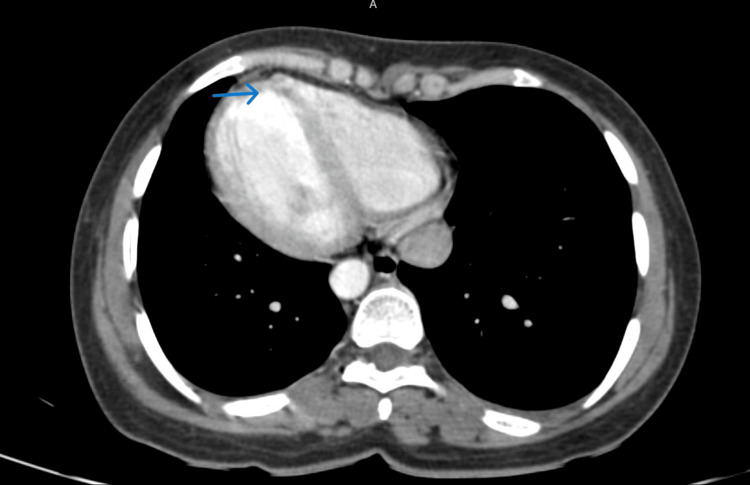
Axial late arterial phase contrast CT scan of the abdomen in lower thoracic sections shows the cardiac apex on the right side CT: computed tomography
